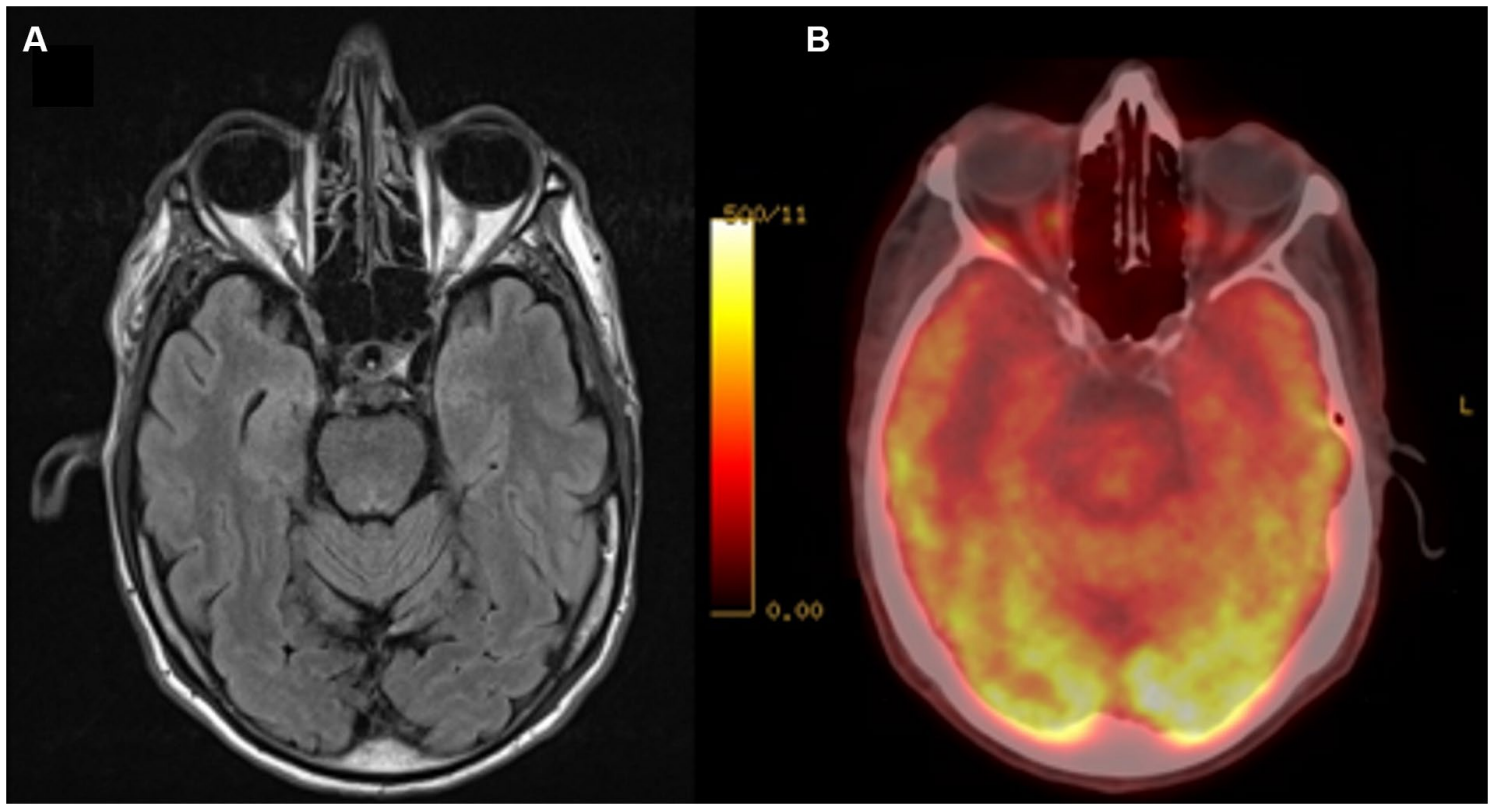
Neuroimaging Exams. (A) Brain MRI, FLAIR sequence; (B) Brain PET/CT with 18F-FDG. A decrease in glycidic metabolism is observed at the bilateral anterior temporal level.
